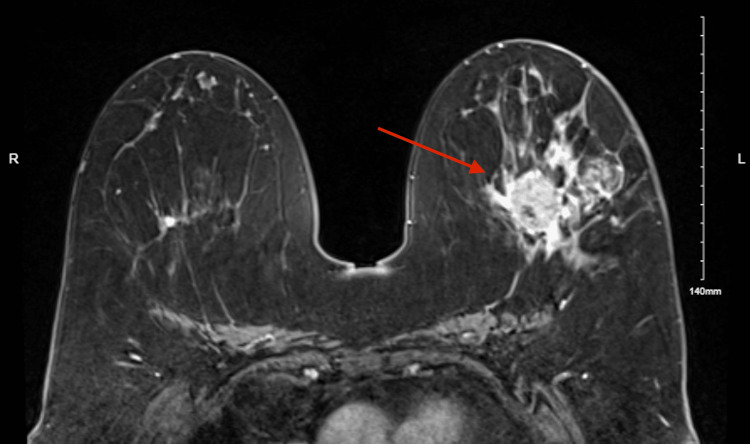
Pre-treatment contrast-enhanced breast MRI This image depicts a contrast-enhanced breast MRI that was obtained before the initiation of treatment. The red arrow indicates a large, irregular mass with spiculated margins in the left breast. MRI: magnetic resonance imaging
